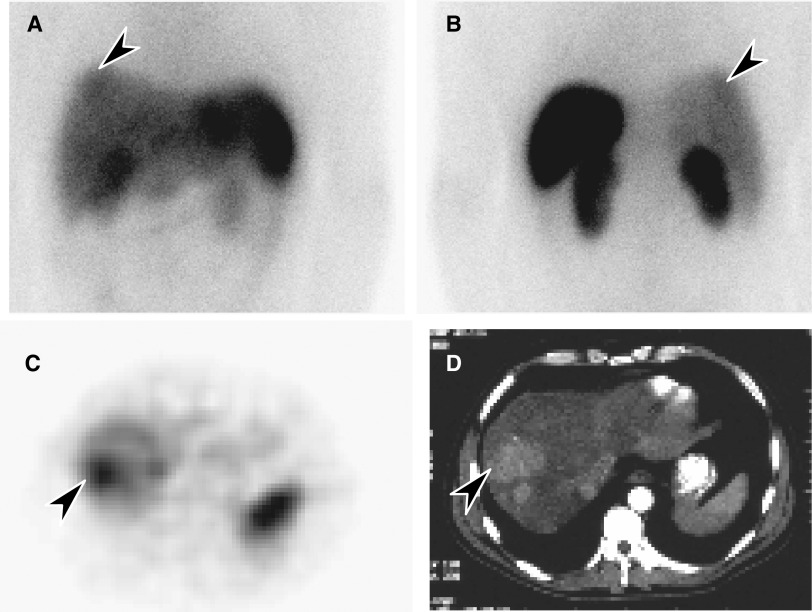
(A) Octreotide scintigraphy (4-h image) showing uptake in an HCC in the dome of the right lobe of the liver (arrow): anterior image; (B) posterior image; normal octreotide uptake in spleen and kidneys; (C) SPECT transaxial section through the upper abdomen showing increased uptake in the HCC (arrow); and (D) the hepatoma in a corresponding CT scan slice (arrow).
